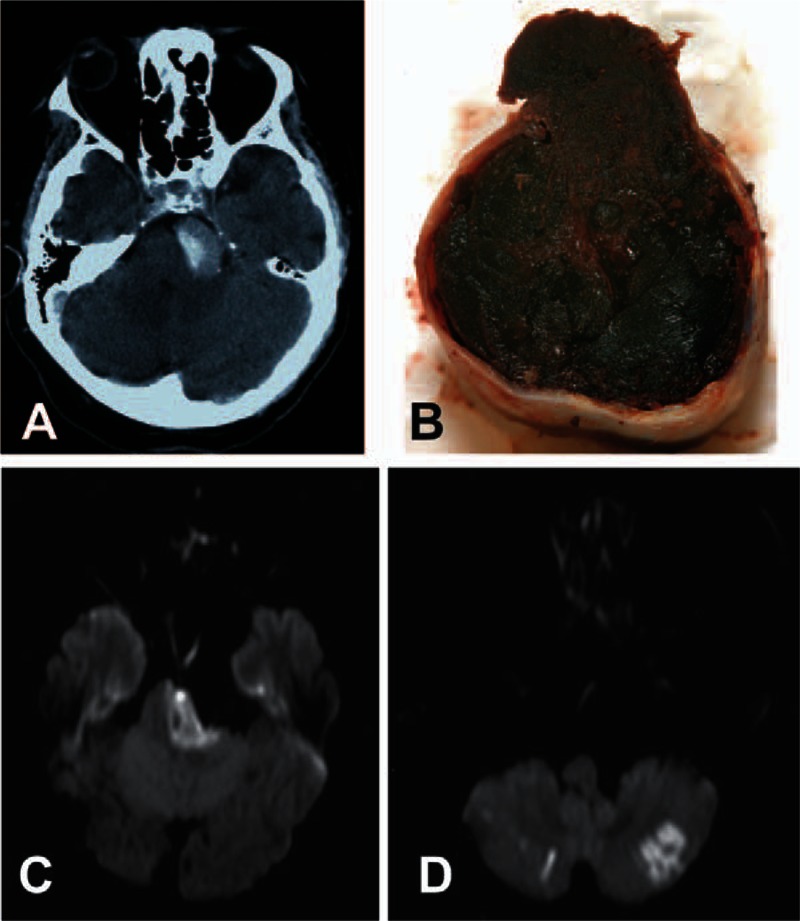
Thrombosis and stroke in dolichoectasia. (A) Axial CT angiogram revealing thrombosis within the dilated basilar trunk of a vertebrobasilar dolichoectasia (VBD). (B) Pathological examination showed multilayer acute-on-chronic thrombi. Only a small residual lumen could be detected. Rupture site (upper segment of the image) was located at the same level as the thrombus. (C) Axial diffusion-weighted MRI showing infarction of the left pons with pontine compression by the dolichoectatic basilar trunk. (D) Diffusion-weighted MRI with infarction in both cerebellar hemispheres. CT = computed tomography, MRI = magnetic resonance imaging, VBD = vertebrobasilar dolichoectasia.
